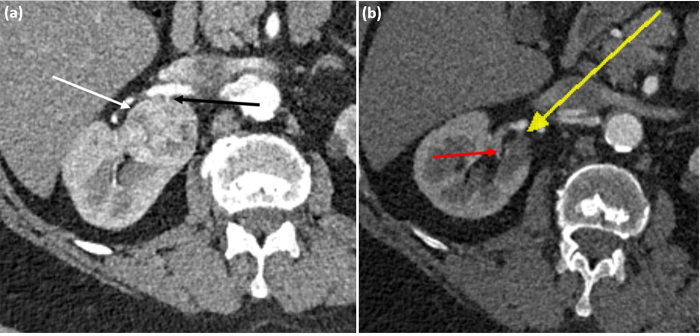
(a) Computed tomography (CT) scan showing renal mass (white arrow) with a small enhancing feeding vessel (black arrow) arising from renal artery. (b) CT scan of right kidney at 11 months after the initial presentation demonstrates the renal lesion had largely regressed (red arrow) and the feeding vessel no longer enhanced on arterial phase imaging (yellow arrow).
